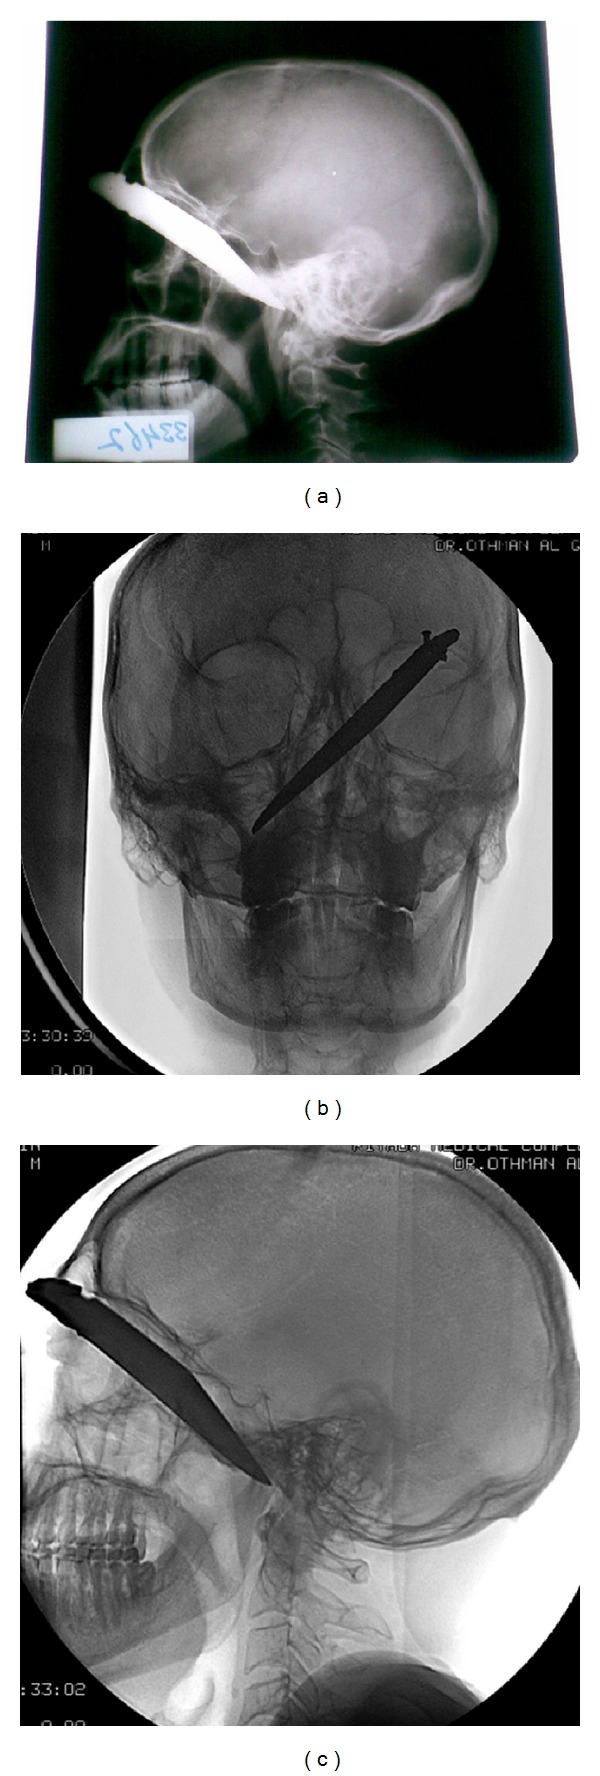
((a), (b), and (c)) Radiographic images describing the route of the knife and the tip reaching the infratemporal region across the midline.
